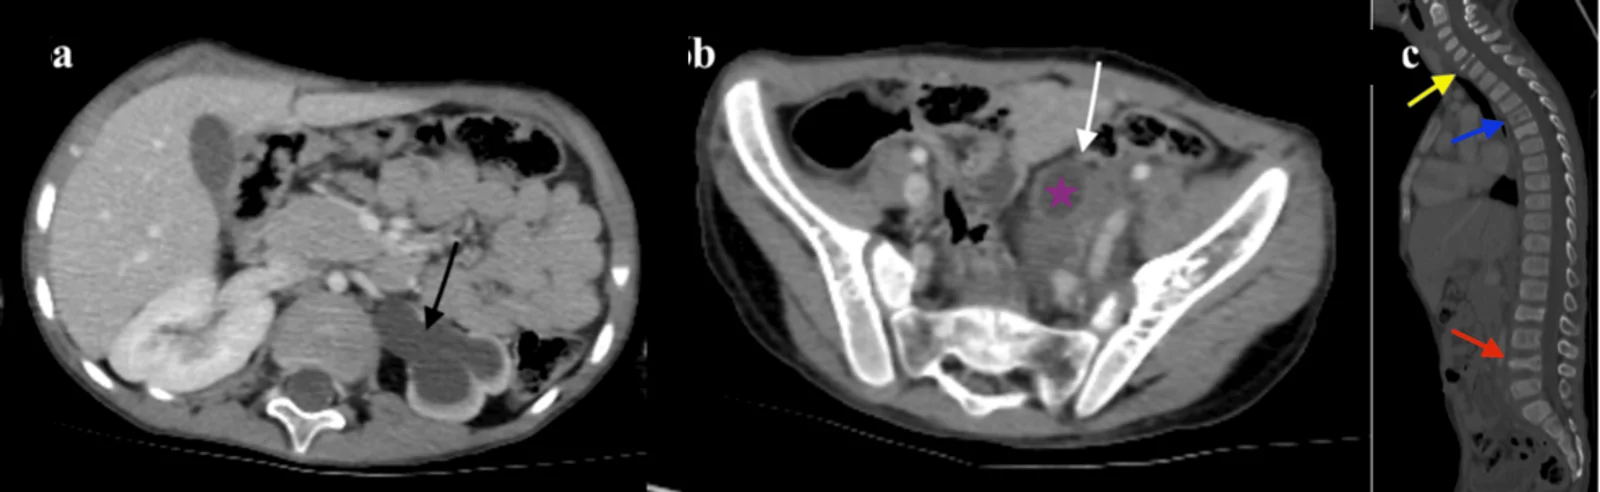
Imaging findings during follow-up. (a) Axial contrast-enhanced CT image showing persistent left ureterohydronephrosis (black arrow). (b) Axial contrast-enhanced CT image showing the residual retroperitoneal tumor tissue (white arrow) adjacent to the dilated left ureter (purple asterisk). (c) Sagittal bone-window reformation of the entire spine showing progression of the L4 compression fracture (red arrow) and a new dorsal lytic lesion (blue arrow), with an unchanged cervical compression fracture (yellow arrow), compared with the initial examination (Figure 2d).
